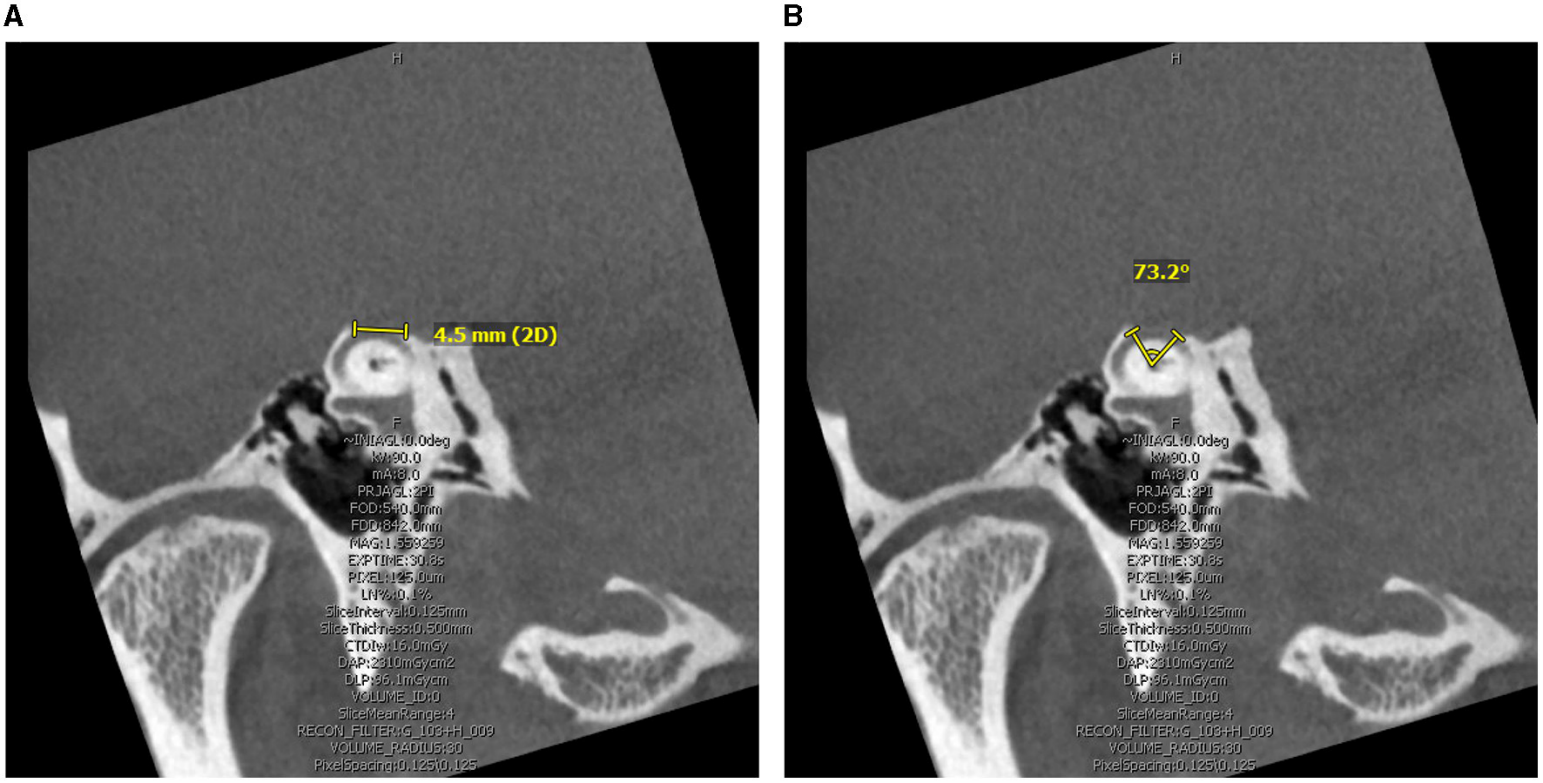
Computed Tomography superior canal dehiscence (SCD) measurement in the Pöschl view. (A) Linear length measurement obtained as the linear distance between the two ends of the SCD. (B) Arc length measurement obtained by radii and angle measure approximating defect center by the arcuate artery.
